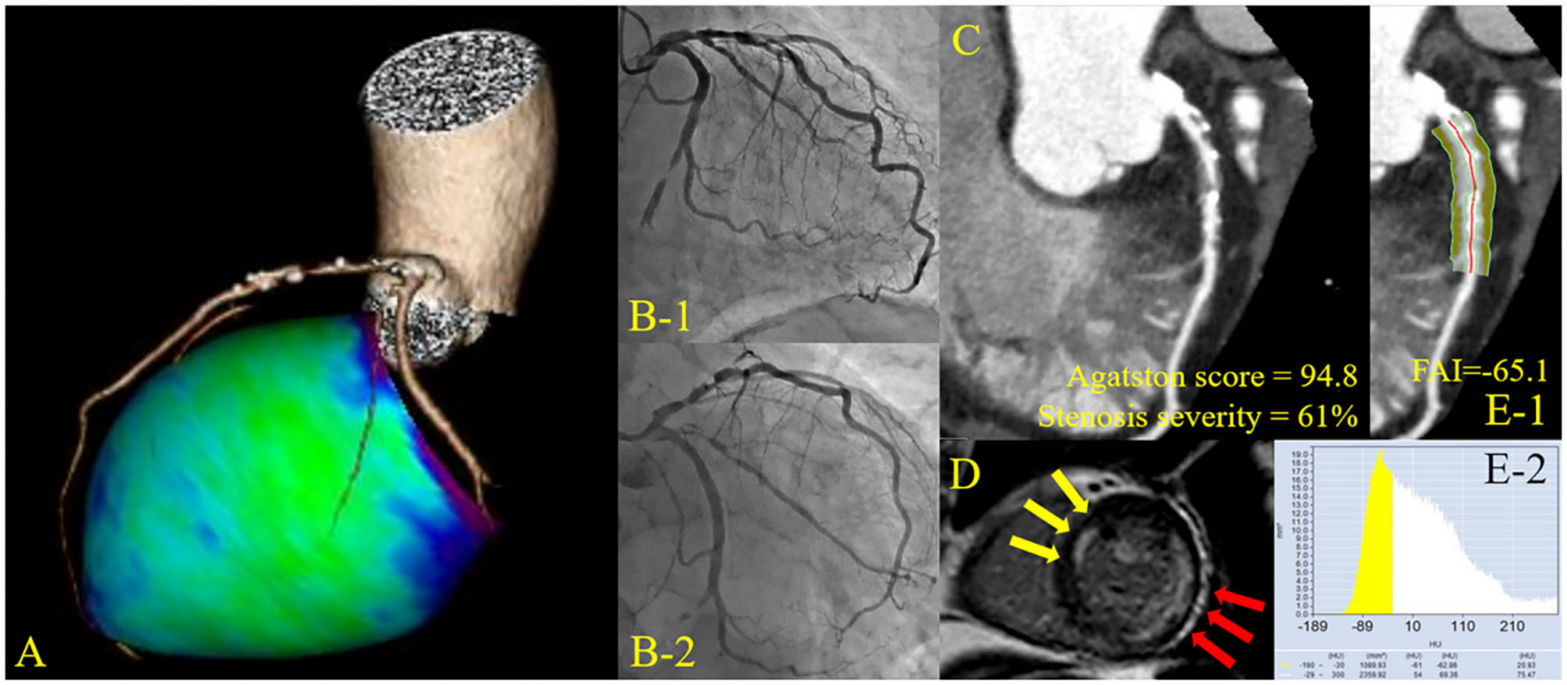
Representative cardiac images of a patient with non-infarct-related unrecognized myocardial infarction. A 72-year-old man with a history of diabetes mellitus. (A) Three-dimensional reconstruction of the coronary artery. (B) Pre-percutaneous coronary intervention (PCI) angiogram reveals 99% stenosis in the left circumflex artery #13 (culprit lesion) and 75% stenosis in the left anterior descending artery #6 (non-culprit lesion). (C) Curved planar reconstruction view with Agatston score and computed tomography-derived stenosis severity. (D) Delayed enhancement cardiac magnetic resonance imaging shows infarct-related late gadolinium enhancement (LGE) in the lateral territory and non-infarct-related LGE in the anterior territory. (E) Peri-coronary fat attenuation index (FAI) at the proximal 40 mm segment of non-infarct-related vessel (left anterior descending artery) was traced.
